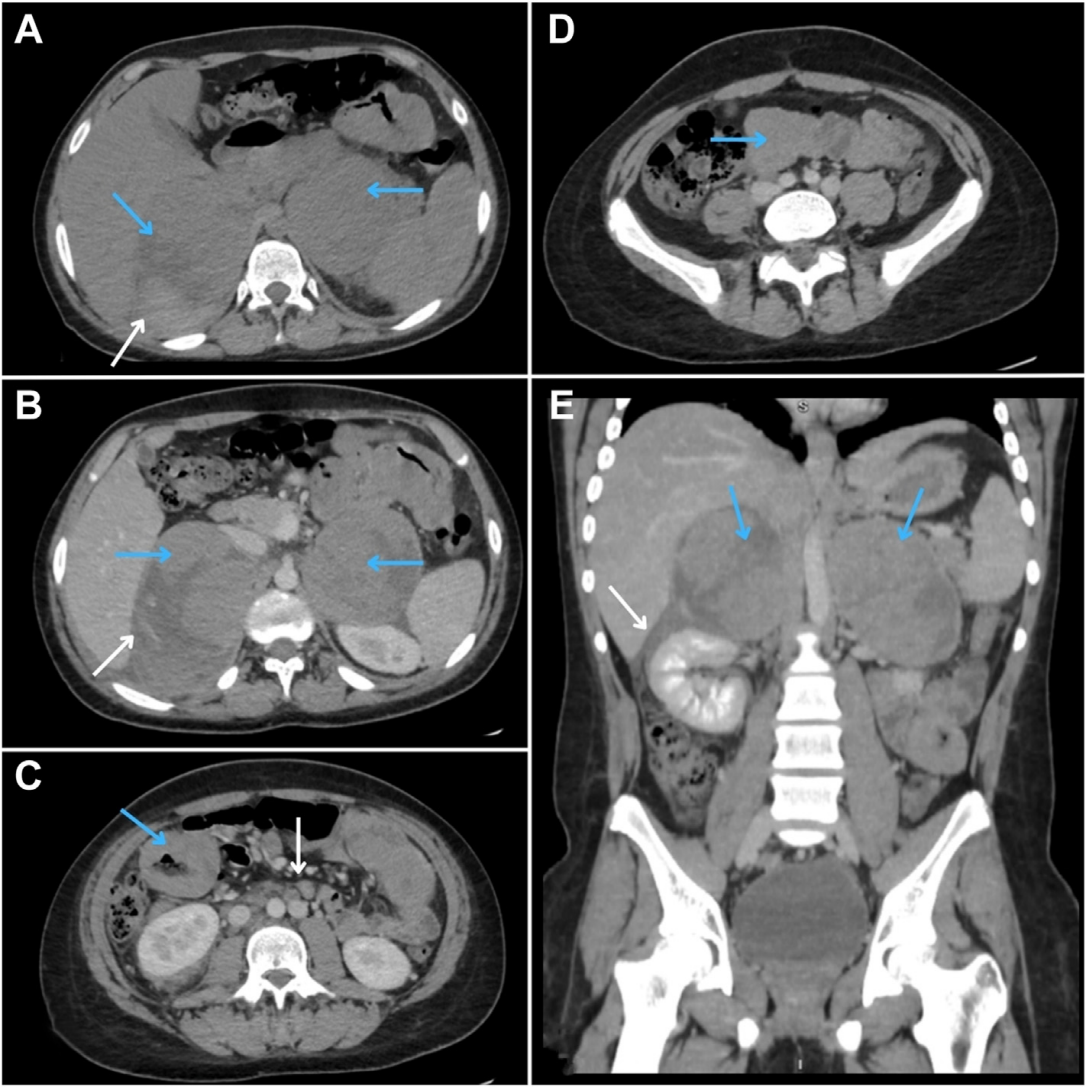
Axial and coronal abdomen computed tomography (CT) scan with and without intravenous contrast. *A*, Axial CT image showed a large, well-defined heterogeneous collections (hematomas) in both adrenal glands (blue arrows) with peripheral dense nodules of soft tissue density (white arrows). *B*, Postcontrast imaging revealed enhancement of the peripheral dense regions of the hematomas (blue arrows) and active contrast extravasation within the right adrenal hematoma (white arrow). *C, D*, Diffuse enhancing wall thickening of small bowel loops was evident (blue arrows), alongside multiple enlarged para-aortic lymph nodes (white arrows). A duplicated inferior vena cava was observed as an incidental finding. *E*, Coronal CT images displayed bilateral adrenal hematomas (blue arrows) with peripheral enhancing nodules and minor extension into the perinephric space on the right side (white arrow).
